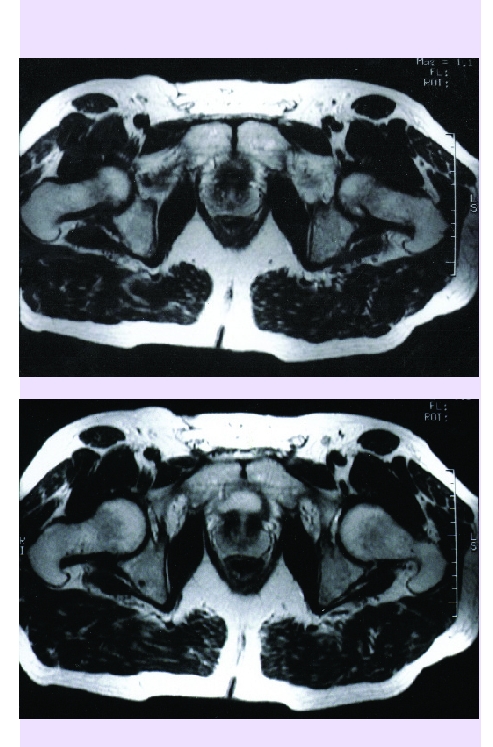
MRI before (a) and after (b) spongioclasia and instillation of preparation (hip arthrosis) at every 3 months.
